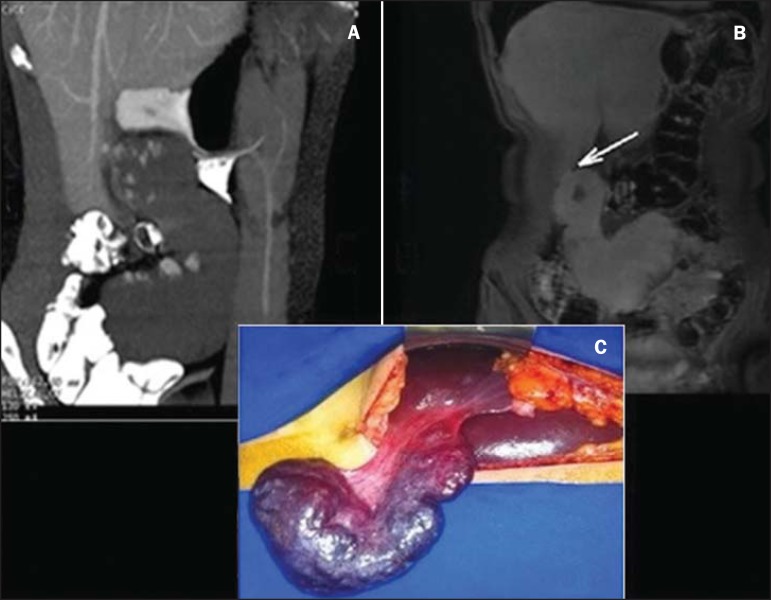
A: Contrast-enhanced total abdominal CT (oral and intravenous
